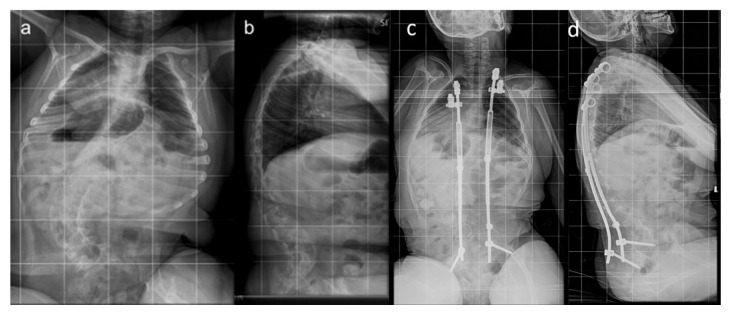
Eight-year-old male VEPTR^TM^ patient EOS secondary to GMFCS V cerebral palsy. Patient had undergone five lengthening procedures by four years post-implantation. He did not experience any post-operative complications and his five-year follow-up EOSQ-24 total score was 61/100. (a) Pre-operative posteroanterior radiograph demonstrating 72-degree scoliosis. (b) Pre-operative lateral radiograph. (c) Four-year post-implantation anteroposterior radiograph. (d) Four-year post-implantation lateral radiograph.
